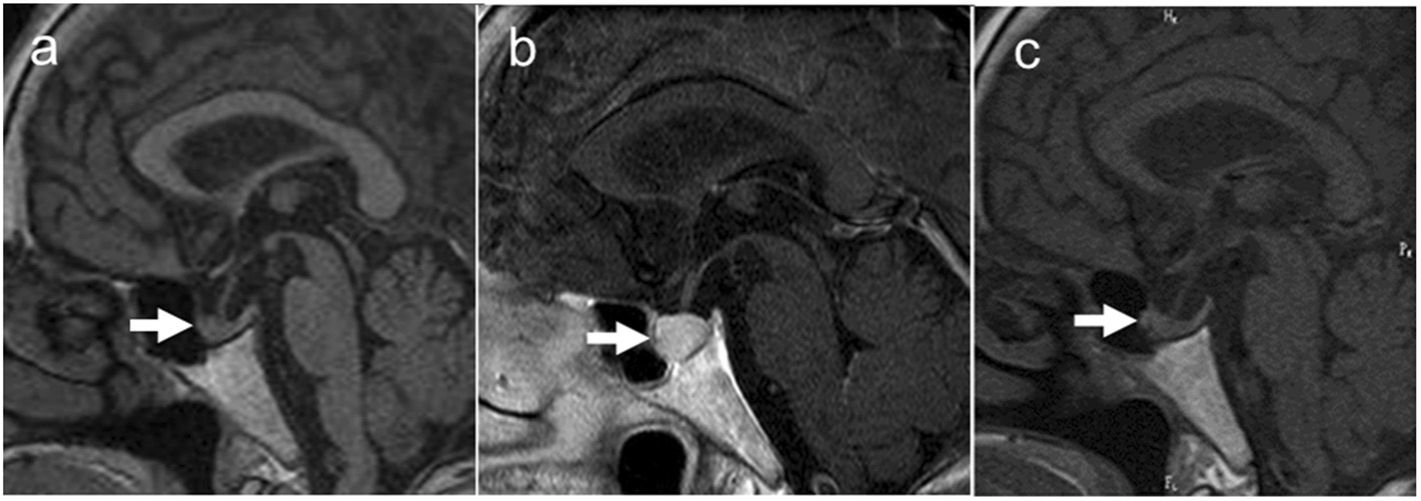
Head magnetic resonance imaging. White arrows indicate the pituitary gland. (a) One year before. Normal-sized pituitary gland. (b) Time of diagnosis. Pituitary gland swelling but keeping signal of posterior lobe of pituitary. (c) After one course of chemotherapy. Pituitary gland swelling improved.
